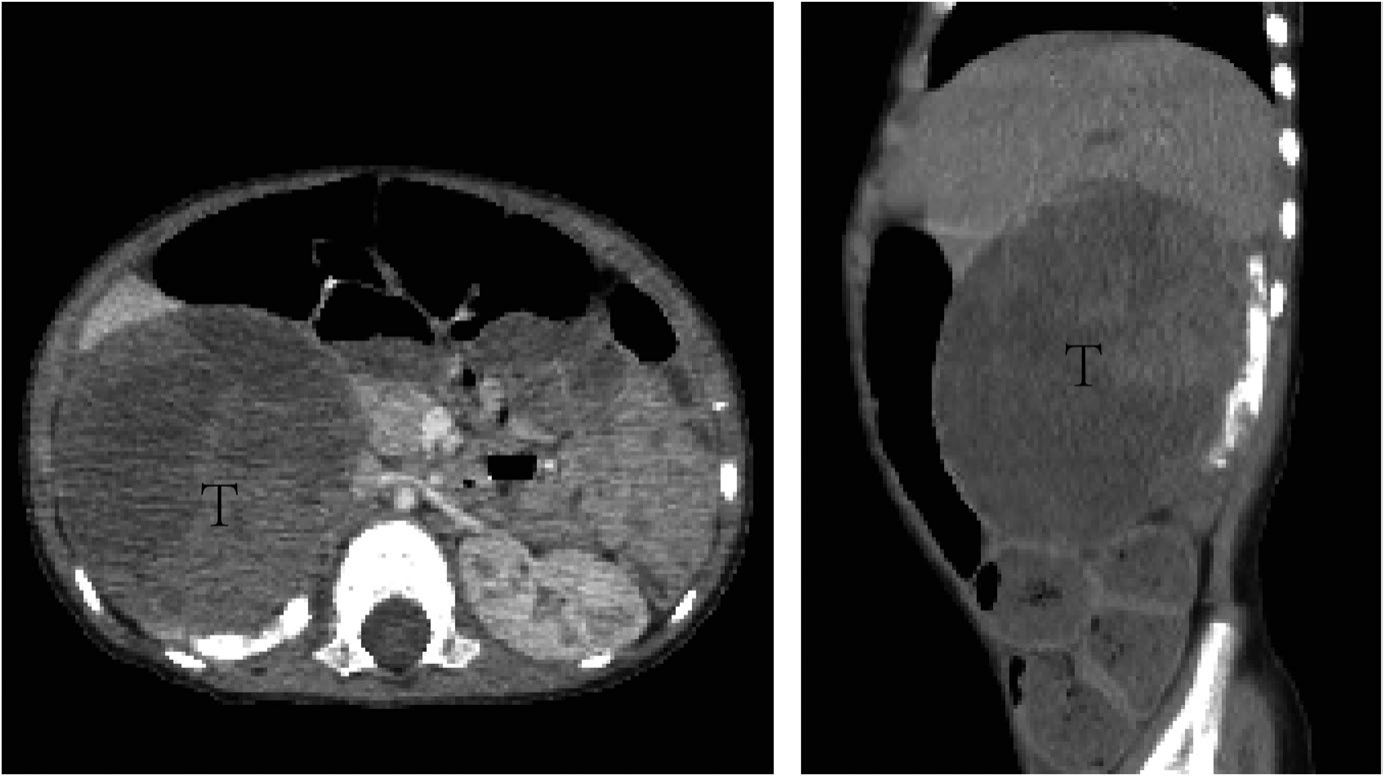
Contrast‐enhanced CT showing the 7.1 cm ×8.0 cm ×7.6 cm sized heterogeneous enhancing mass in the upper region of the right kidney. Left: transverse view of the tumor, Right: sagittal view of the tumor
